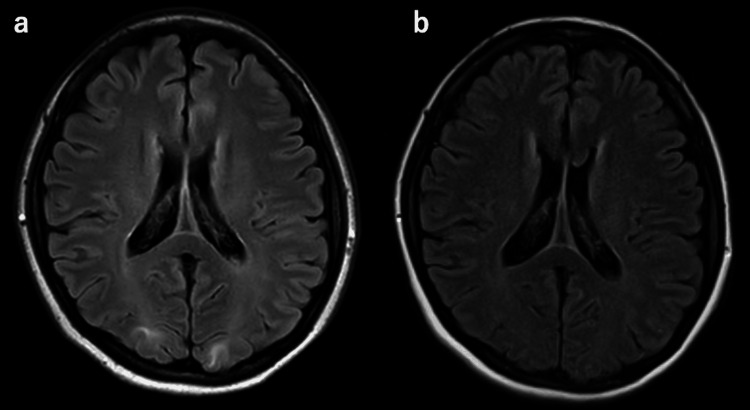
Axial FLAIR MRI (1.5 T; repetition time, 11,000 ms; echo time, 140 ms) on day 3 (a) and day 17 (b) (1.5 T; repetition time, 10,000 ms; echo time, 102 ms). (a) Partial resolution of lesions after 3 days. (b) Complete resolution of lesions by day 17. FLAIR, fluid-attenuation inversion recovery; MRI, magnetic resonance imaging.
